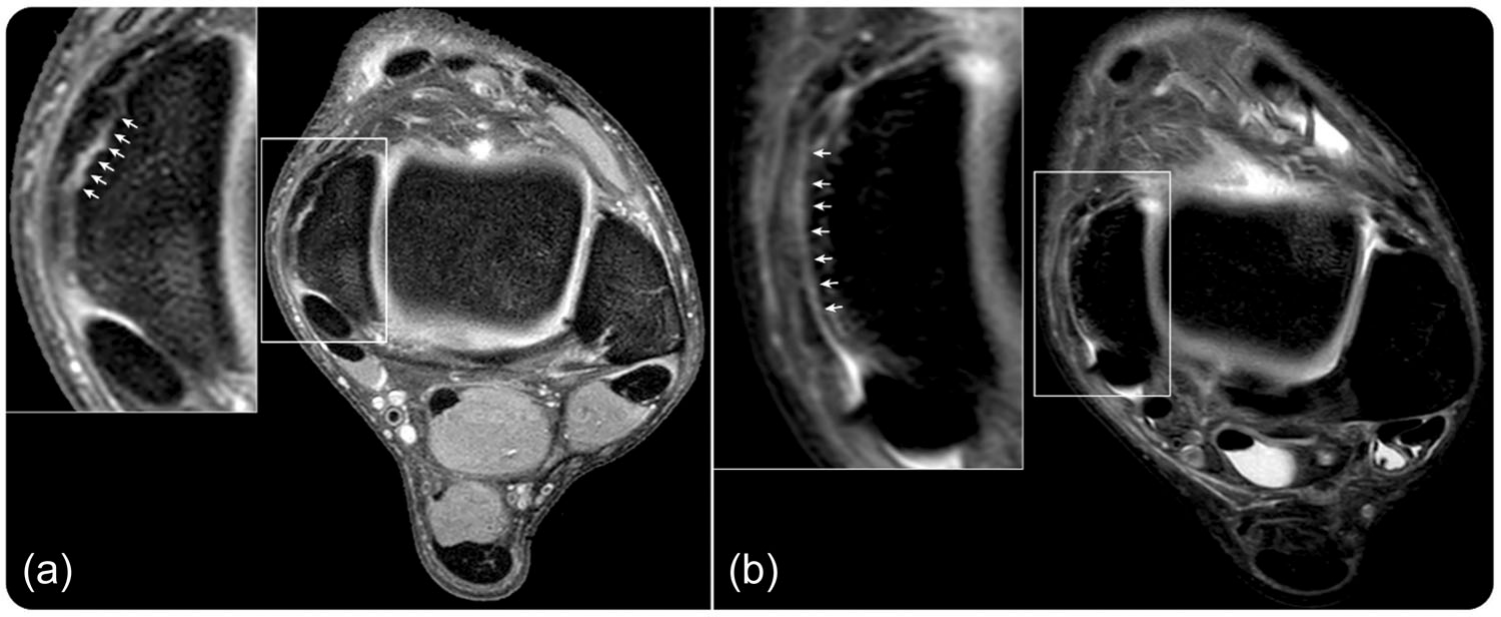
T2 Axial MRI sequence of the ankle joint, showcasing a complete medial sleeve rupture with visible fluid between the sleeve and medial malleolus.
